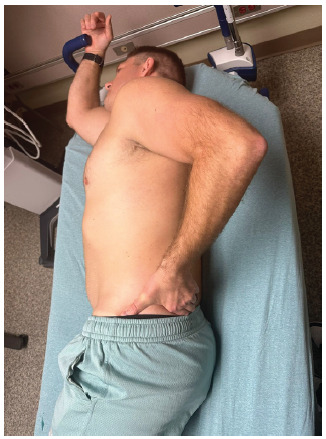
Volunteer in modified Crass position for the supra-short ultrasound protocol.
